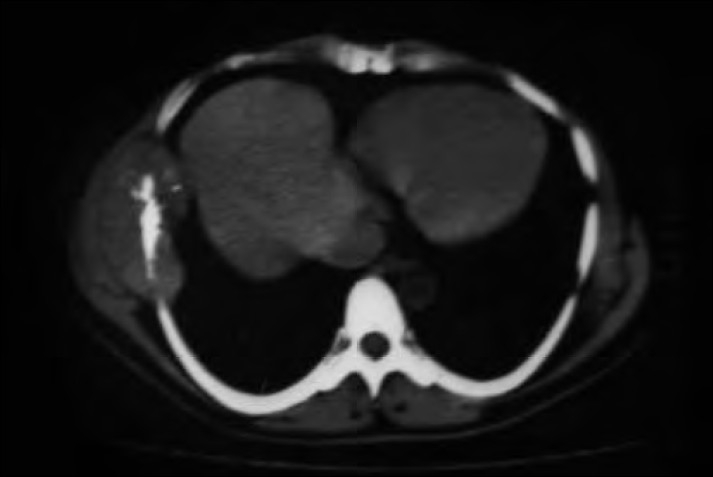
Axial CT image showing a lobulated, soft tissue density mass with calcification and sclerosis, and is seen destroying the rib
